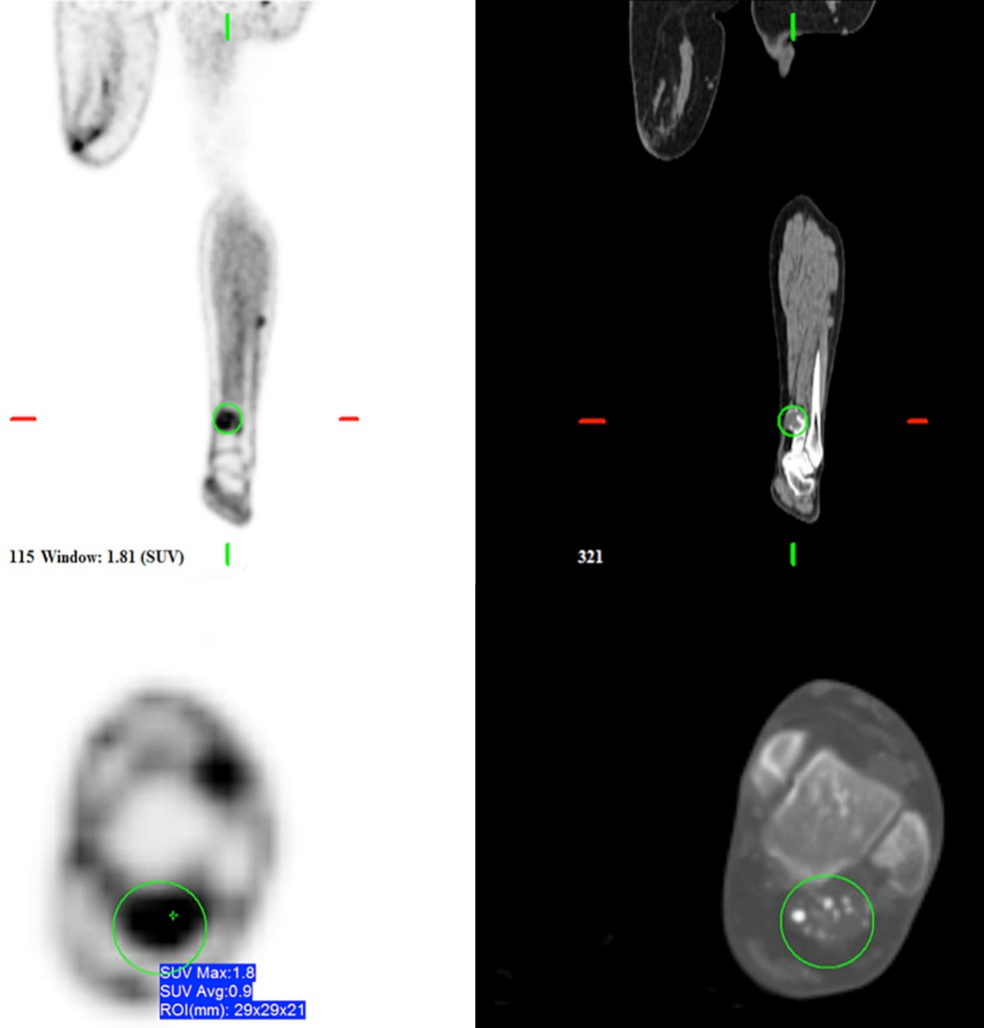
PET and CT coronal and axial images of the bilateral lower extremities demonstrating low-grade uptake (SUV: 1.8) lesions in the left lower leg CT: computed tomography, PET: positron emission tomography, SUV: standardized uptake value
